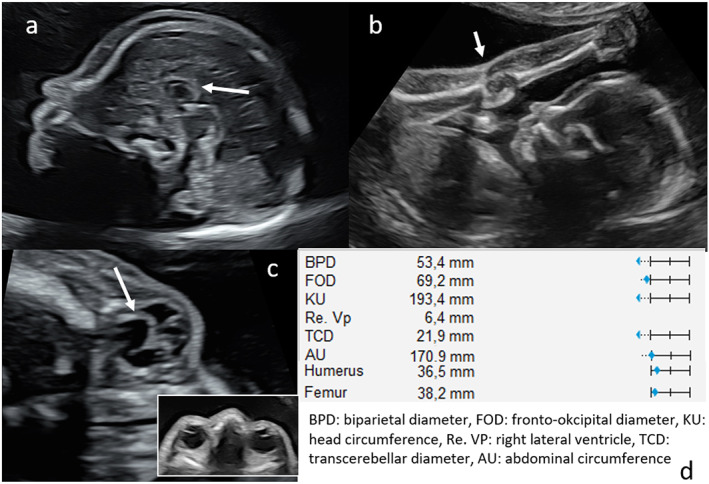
Sonography at 24 weeks of gestation.* (a) Dysplastic short corpus callosum (arrow), hypoplastic brain stem (b) legs in fixed extension at the knees (arrow) in front of fetal body, (c) intraocular anomaly (retinal detachment, arrow), (d) fetal biometry measurements (in centiles).
